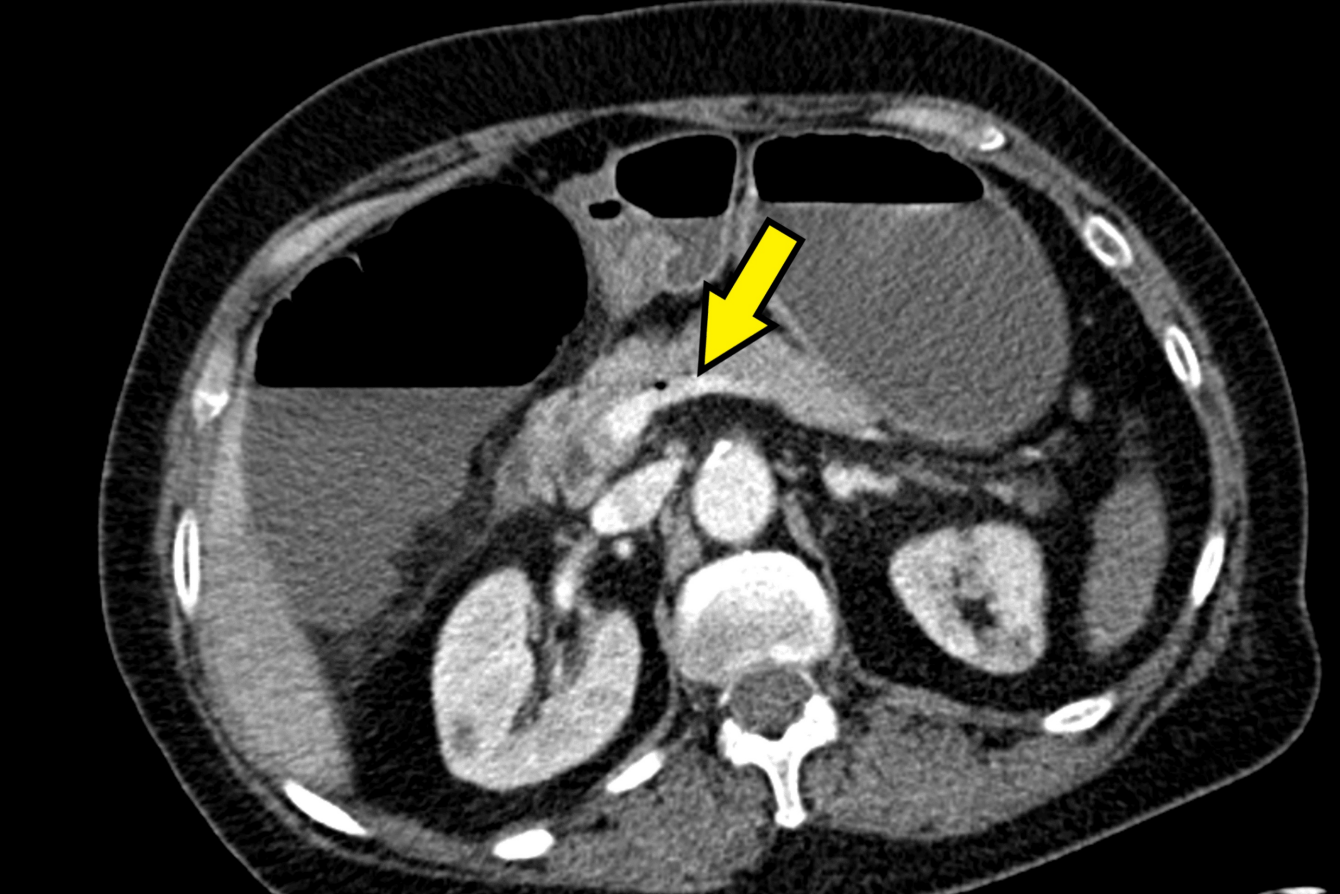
Contrast-enhanced abdominopelvic CT scan Yellow arrow: Signs of mesenteric ischemia with intestinal pneumatosis
